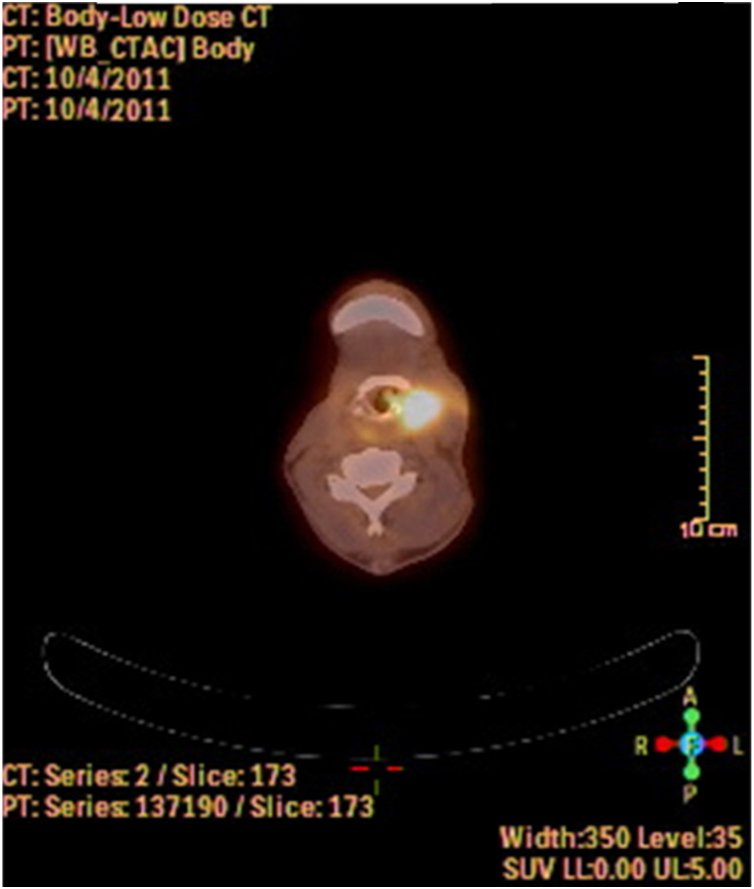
A PET-CT image showing a dense hypermetabolic involvement in larynx. Appearance of locoregional recurrence following partial laryngectomy.
